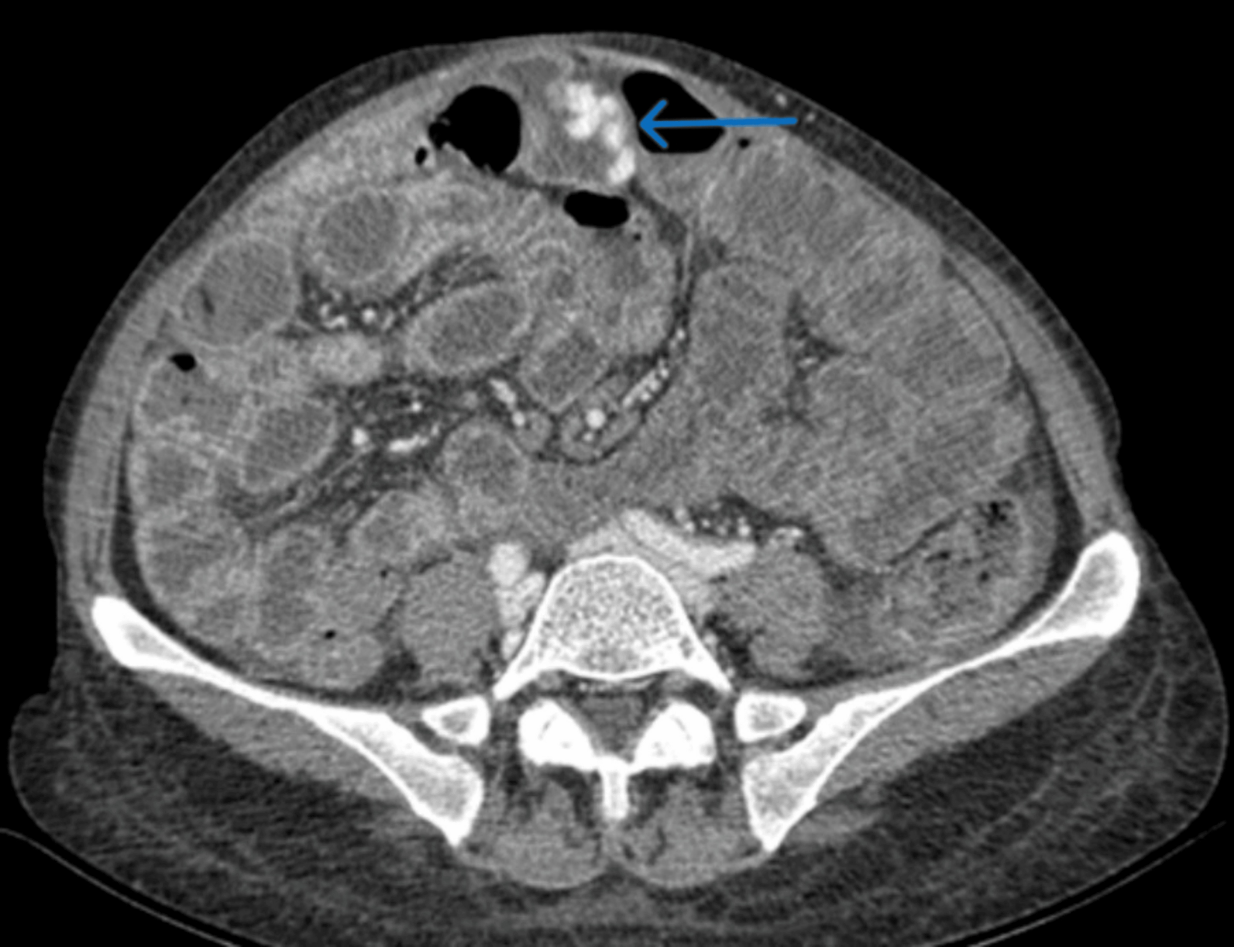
Portal-phase abdominal CT showing jejunal varices (blue arrow)
